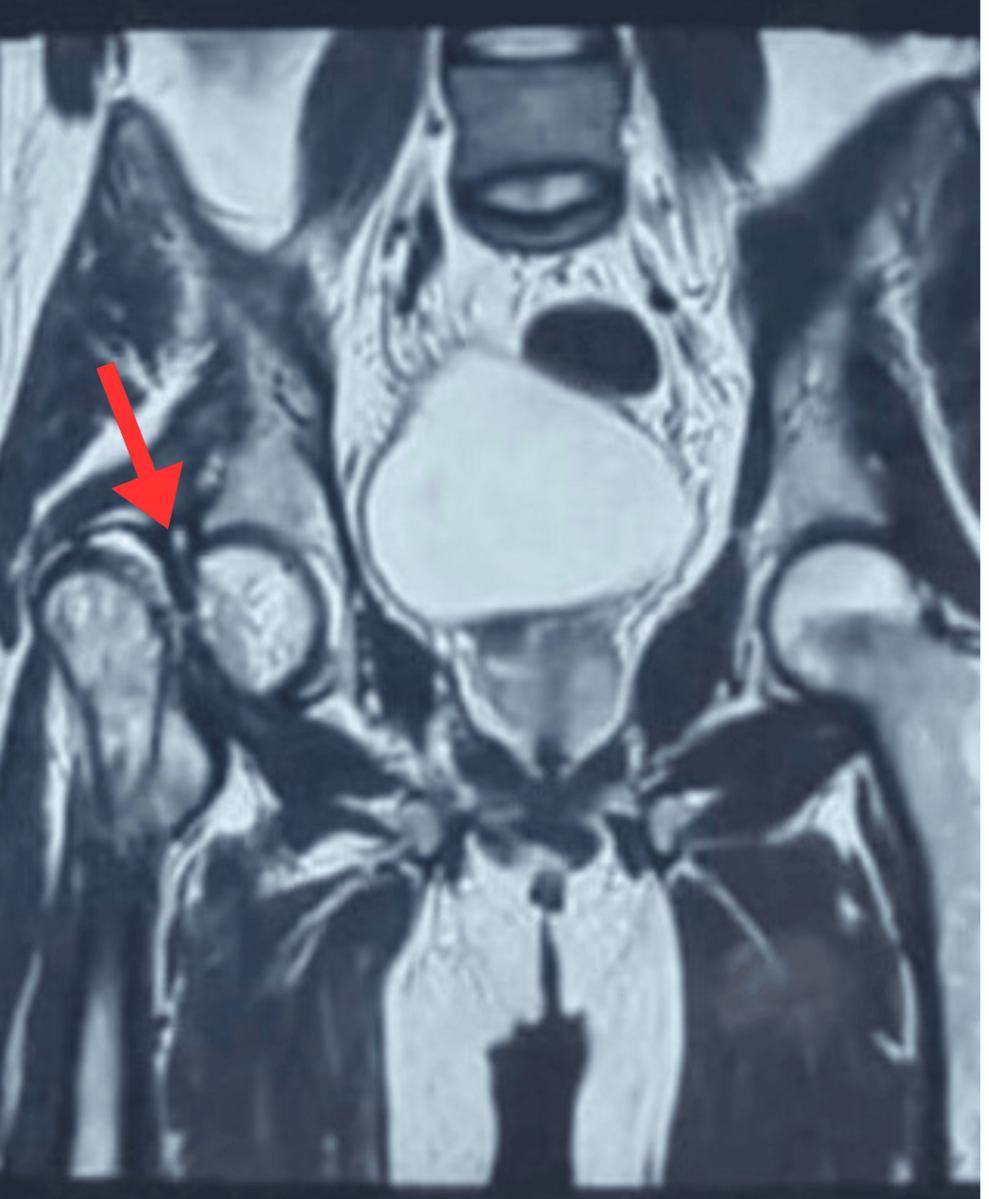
Sagittal section of MRI showing non-union of neck of femur fracture The red arrow indicates non-union of the femoral neck and partial resorption of neck.
